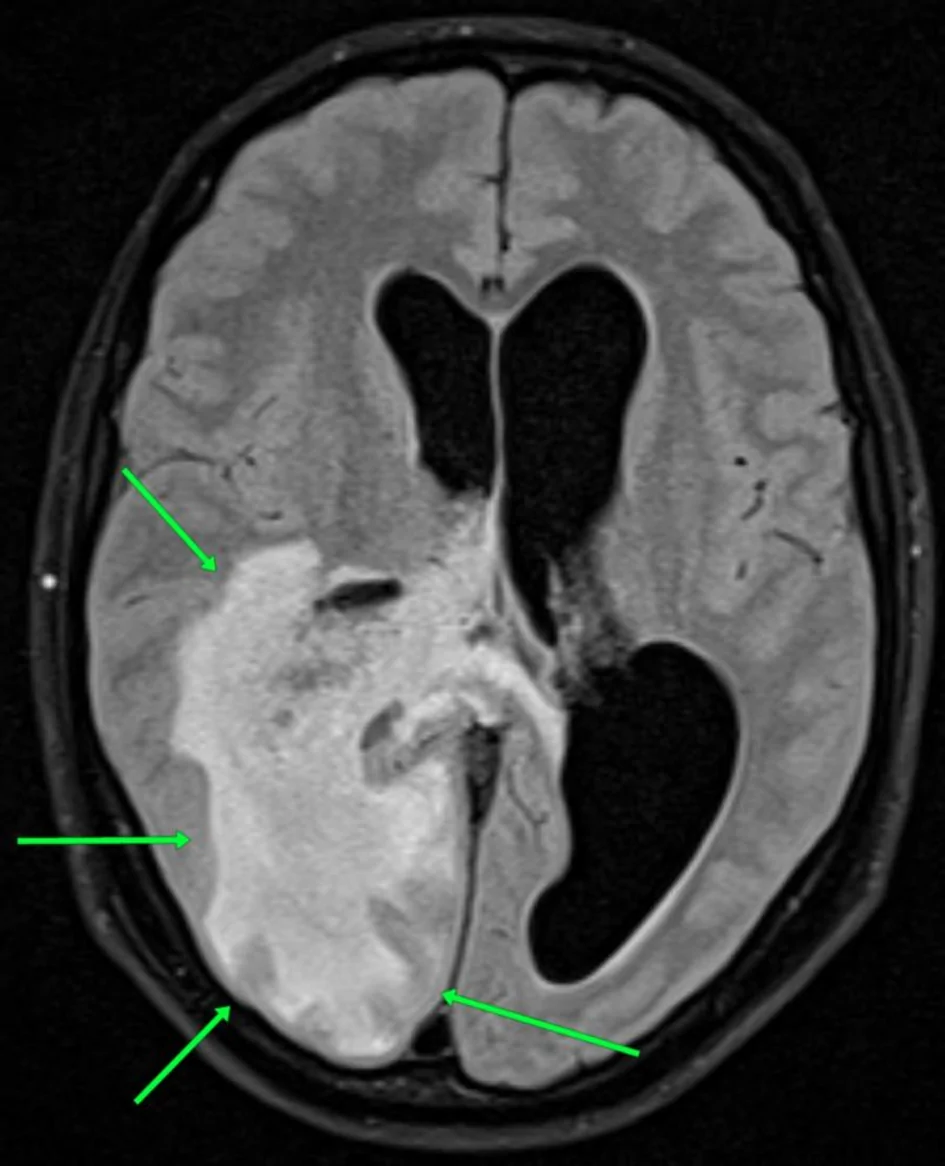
Axial T2-FLAIR MRI demonstrating marked periventricular white matter edema involving the adjacent temporal and occipital lobes (green arrows). FLAIR: fluid-attenuated inversion recovery; MRI: magnetic resonance imaging.
